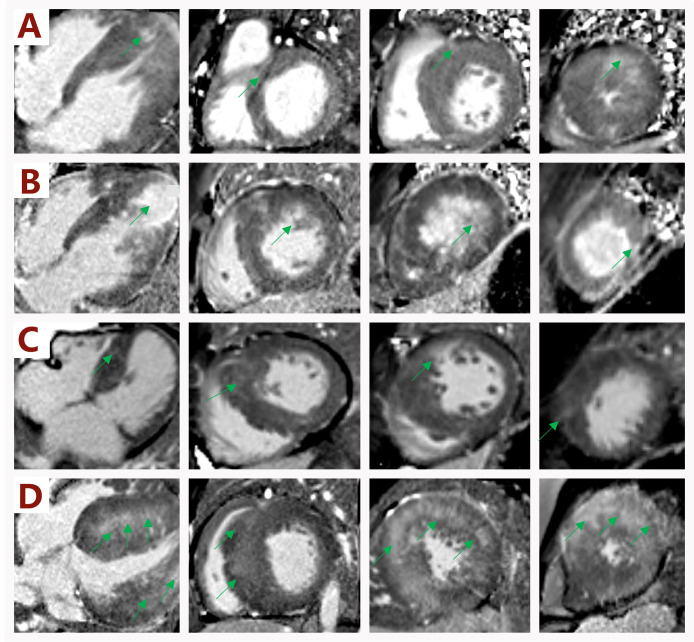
CMR delayed enhancement imaging (LGE-CMR) has demonstrated four typical types of myocardial fibrosis distribution in patients with hypertrophic cardiomyopathy. (A) Apical hypertrophic cardiomyopathy: LGE shows diffuse cloud-like and patchy delayed enhancement from the base to the apical myocardium, with enhancement predominating in the apical portion, suggesting widespread fibrosis. (B) Apical hypertrophic cardiomyopathy with ventricular aneurysm: LGE enhancement is mainly distributed in the mid-segment septum and right ventricular insertion with obvious enhancement of the sub-endocardium of the apical segment and the myocardium of the apical segment, and a ventricular aneurysm is present at the apical segment. (C) Interventricular septal hypertrophic cardiomyopathy: LGE shows multiple patchy delayed enhancements of the myocardium from the basal segment to the middle of the interventricular septum, which is focally striated. (D) Left ventricular diffuse hypertrophic cardiomyopathy: LGE shows multiple segments of the left ventricular myocardium with patchy abnormal enhancement signal shadow. A small area of low signal shadow is seen in the area of enhancement in the interventricular septum, suggesting widespread myocardial fibrosis with local inhomogeneous changes. Green arrows indicate the regions of myocardial fibrosis in different types of hypertrophic cardiomyopathy. Source: images from our hospital’s cardiovascular surgery department from 2022 to 2024. LGE-CMR, late gadolinium enhancement cardiac magnetic resonance imaging.
